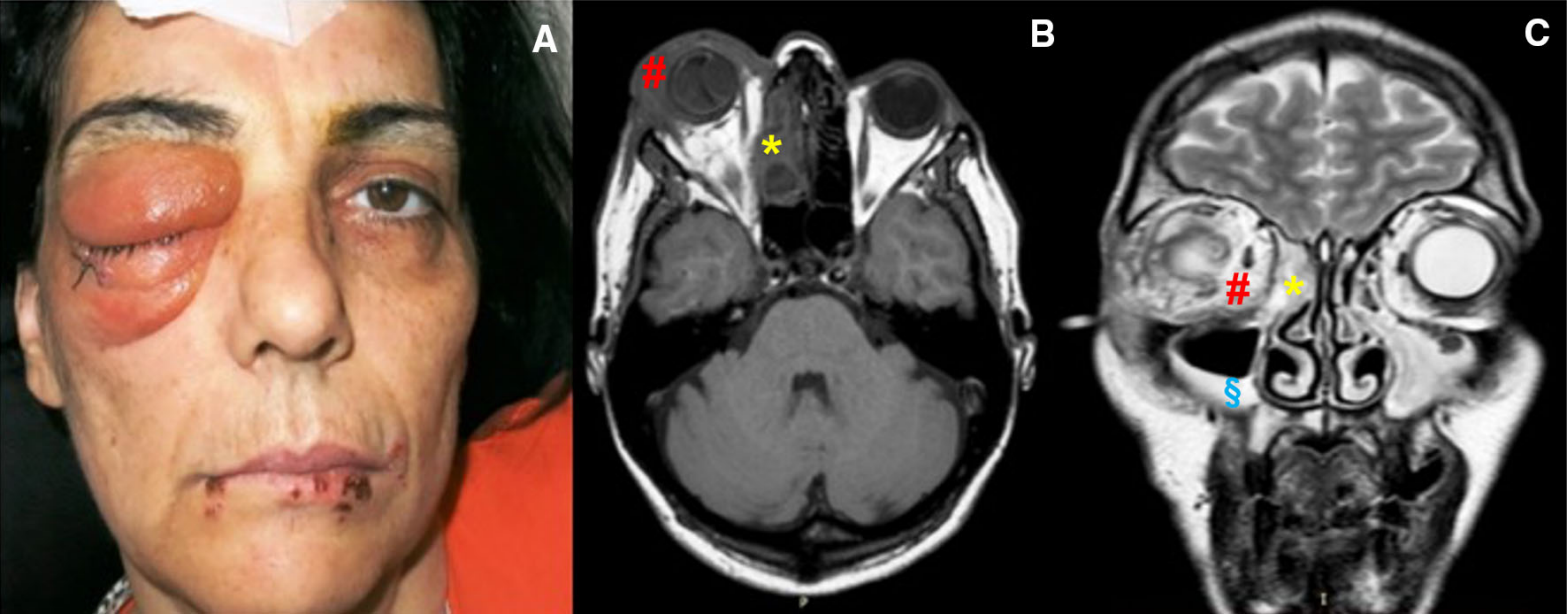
Case 3: Presurgical photo of patient in frontal section (a) and MRI Axial section (b) and MRI Coronal section (c): ethmoid abscess (*), hyper-haemic maxillary sinus mucosa (§), orbital abscess (#)
